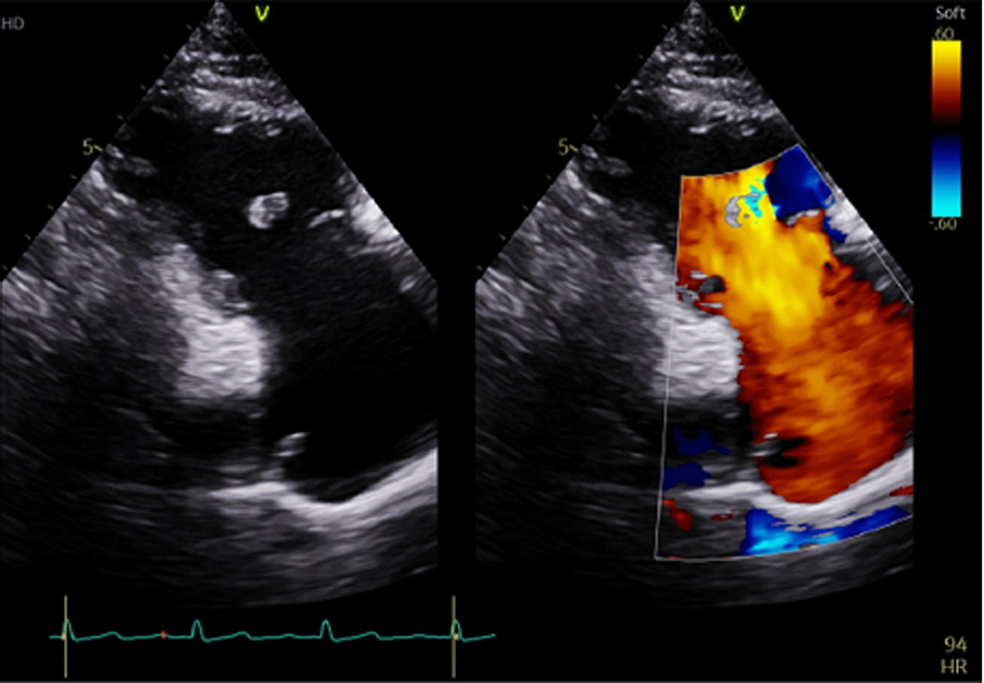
Flail anterior leaflet with torrential tricuspid regurgitation on color Doppler
